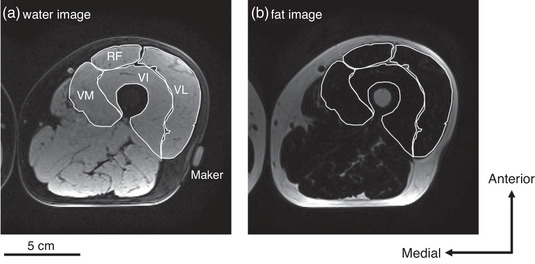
Typical magnetic resonance image examples using fat‐water separated two‐point Dixon imaging: (a) water image and (b) fat image. RF, rectus femoris; VI, vastus intermedius; VL, vastus lateralis; VM, vastus medialis.
